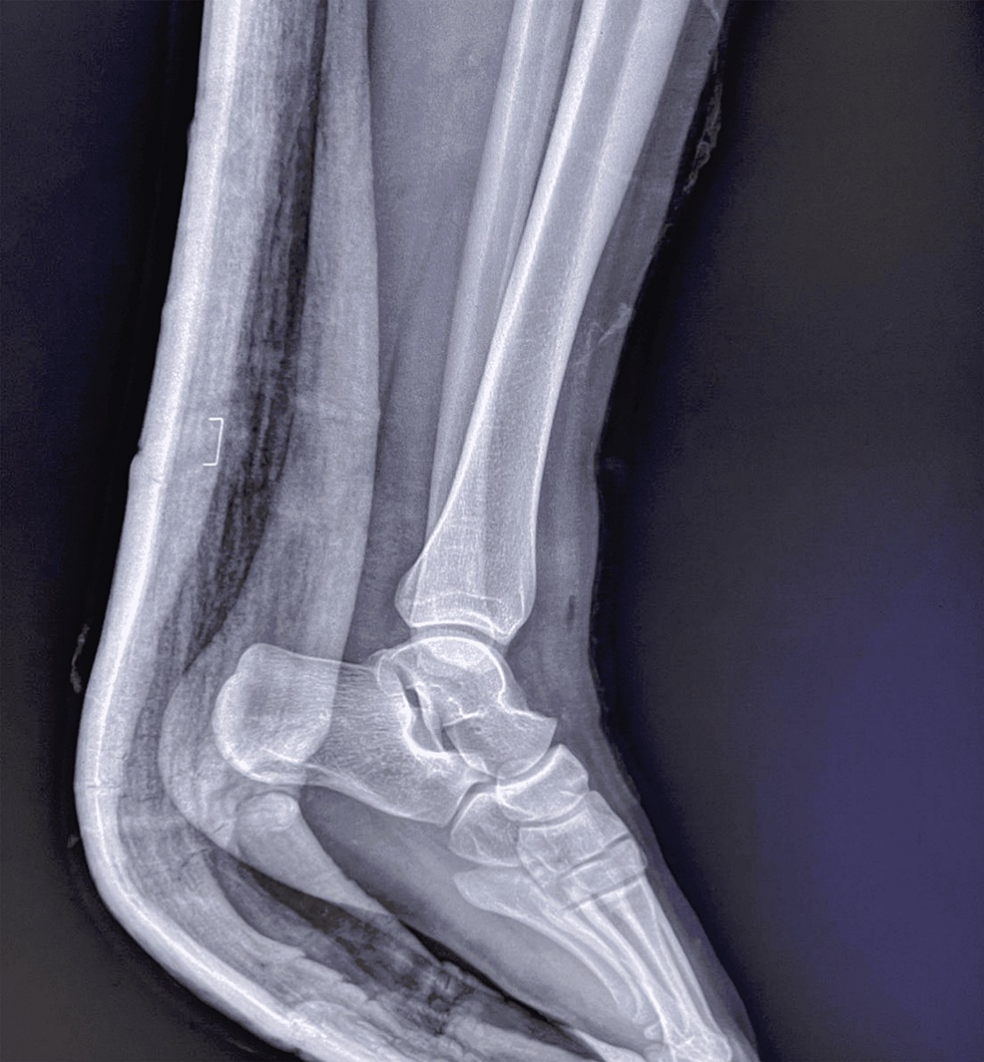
Lateral post-reduction radiographic view of the left ankle.
